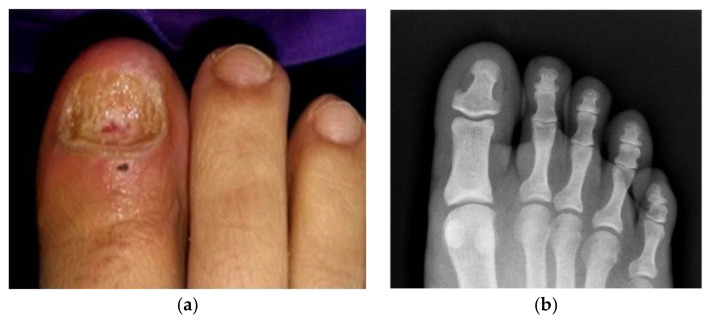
(a) Appearance of nail bed after debridement. The image shows the presence of exudate. (b) Dorsoplantar radiography did not reveal the existence of any lytic image in the distal phalanx of the first toe.
